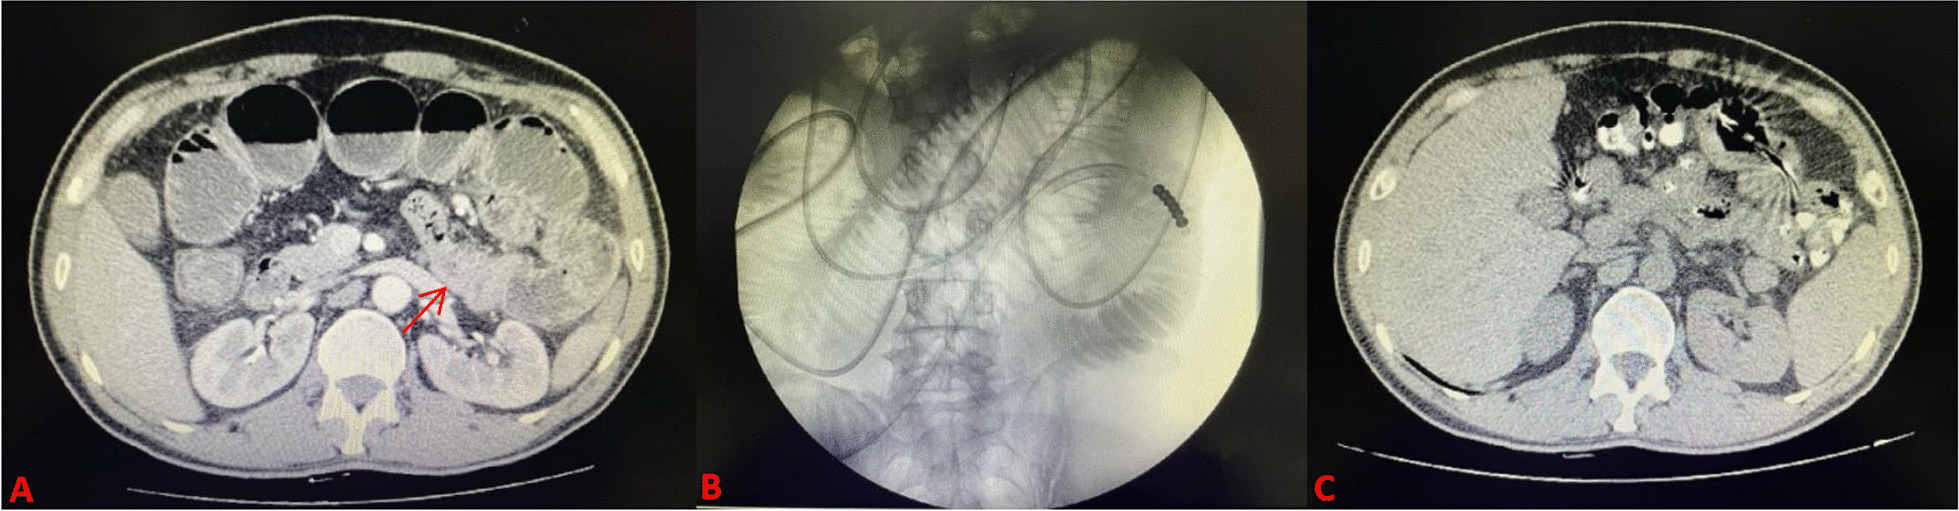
CT image of a patient with malignancy ileus who failed to decompress after NIT insertion. A Before NIT insertion(at the arrow is a tumor); B X—ray of completion of NIT insertion; C Three days after NIT insertion
